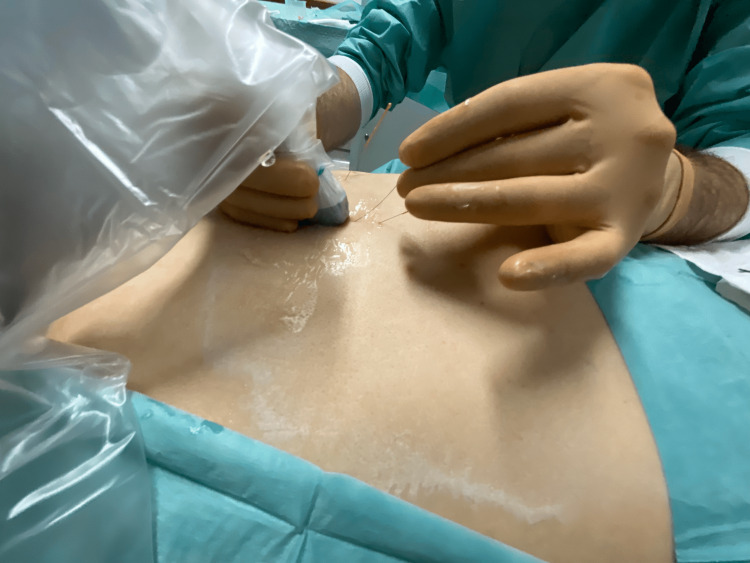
Needle placement under ultrasound guidance Insertion of a needle in the right thoracic parascapular region. The ultrasound probe is protected by a plastic sleeve and sterilized ultrasound gel was used. Image obtained by Pedro Afonso and edited by Helder Cardoso, with patient consent.
